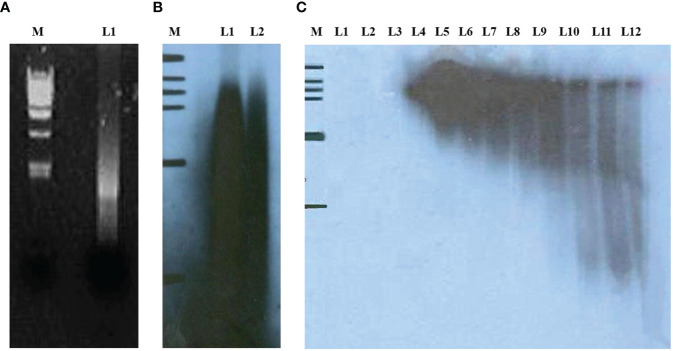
(A) L1 indicates mRNA extracted from 10 lung cancer tissues and mixed in equal amounts; (B) radiographic autoradiographs of the first and second strands of cDNAs after alkaline agarose gel electrophoresis; (C) radiographic autoradiographs of cDNA fragments separated chromatographically and collected by Sepharose CL-2B gel column after non-denaturing polyacrylamide gel electrophoresis.
